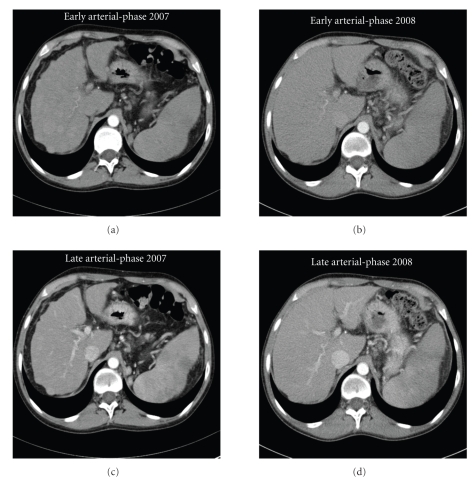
Arterial-phase axial CT images following the administration of contrast medium demonstrate multiple hyperdense nodules at the early arterial phase. Nodules are not visible on unenhanced images.
